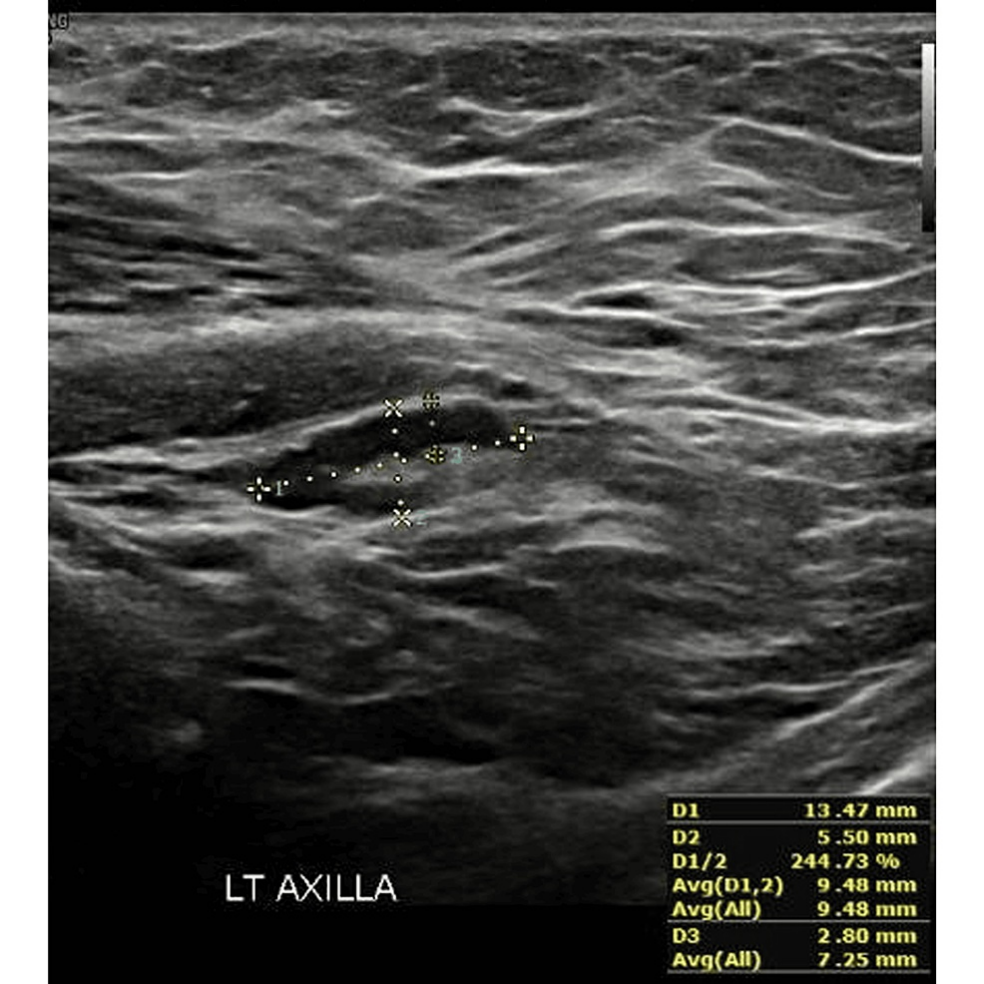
Ultrasound image showing a reactive axillary lymph node - Case A This figure was obtained from the KFUH system (author's own).
